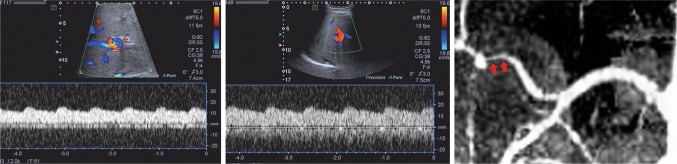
Case meeting category 3 and proven to be HAS on CTA. Male, 34 years old, 3 months after LT due to liver cirrhosis associated with hepatitis B. TPWs were shown on DUS, with RI = 0.29 and SAT = 0.11 s of the right HA (left), and RI = 0.30 and SAT = 0.11 s of the left HA (middle). Although both AST and ALT were normal on the same day, the patient insisted on further examination, and immediate CTA was performed on the same day, which showed HAS stenosis of 95.0% (right red arrow)
